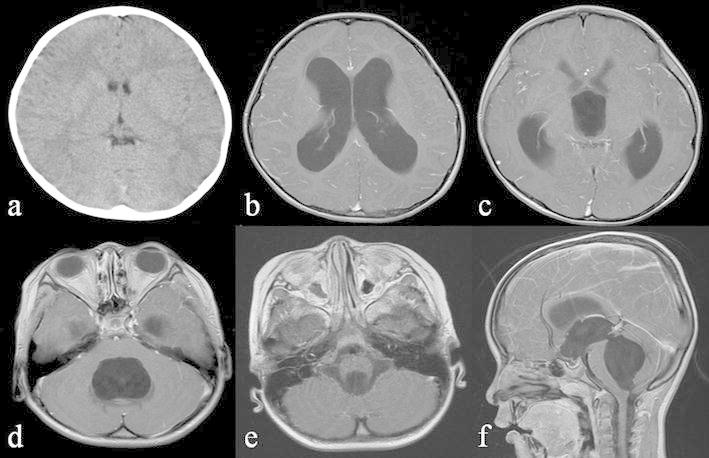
a Computed tomography images obtained to evaluate a head injury 2 years prior to admission, which did not indicate any apparent intracranial abnormality. b–d Contrast-enhanced T1-weighted image (WI) on admission indicating the dilatation of bilateral, third and fourth ventricles. e–f Contrast-enhanced T1-WI (*E* axial, *F* sagittal) indicating no obstructive lesions such as a brain tumor or cerebellar anomaly
